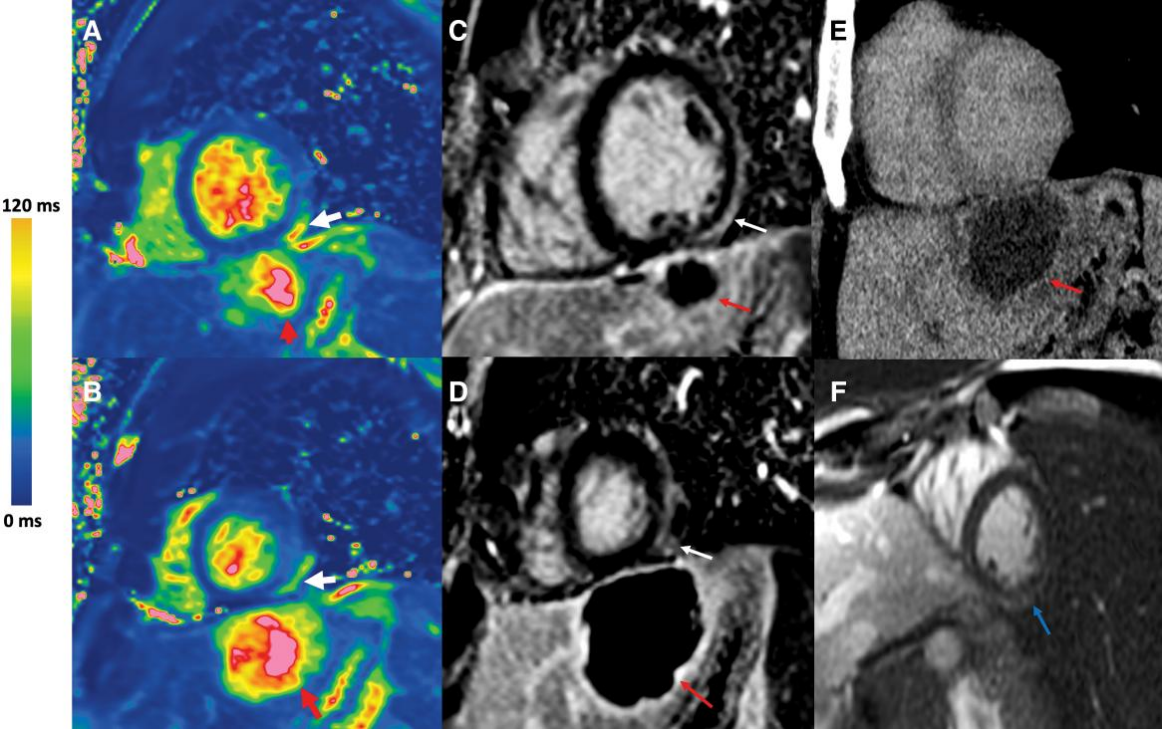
Pyogenic liver abscess with myocarditis. (*A* and *B*) T2 mapping sequence (CMR). Presence of a T2 elevation in the subepicardial region of the inferolateral wall (white arrow). Visualization of the hepatic abscess (red arrow). (*C* and *D*) LGE sequence (CMR). Presence of subepicardial enhancement in the inferolateral region (white arrow). Visualization of the hepatic abscess (red arrow). (*E*) CT scan showing hepatic abscess (red arrow). (*F*) Six-month CMR showing persistent LGE (blue arrow).
